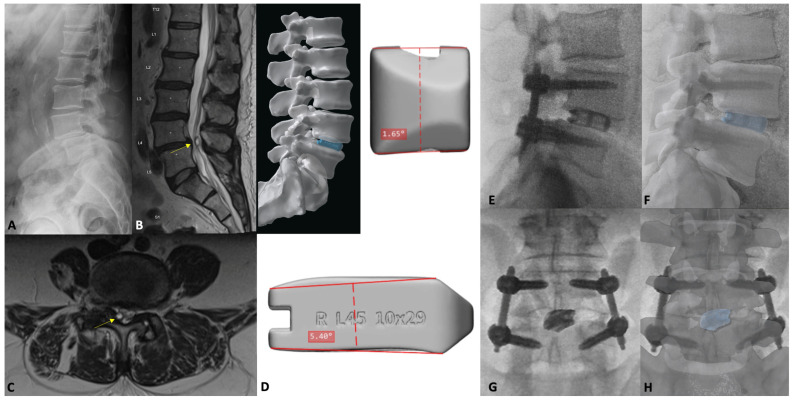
Lateral flexion plain film (A) demonstrating the L4-5 spondylolisthesis and disc height loss. Sagittal (B) and axial (C) T2-weighted MRI depicting the L4-5 facet cyst (yellow arrow) and lateral recess stenosis. Preoperative 3D rendering (D) of the patient’s spine and proposed custom L4-5 interbody (Carlsmed aprevo, Carlsbad, CA, USA). Postoperative lateral (E) lumbar plain film with overlying custom cage rendering (F). Postoperative AP (G) plain film with overlying custom cage rendering (H).
